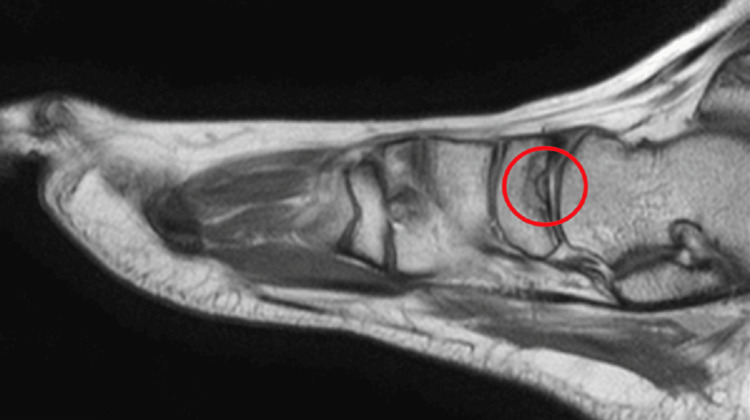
Outside hospital left ankle magnetic resonance imaging showing a subchondral navicular bone fracture with bony edema (circle).
